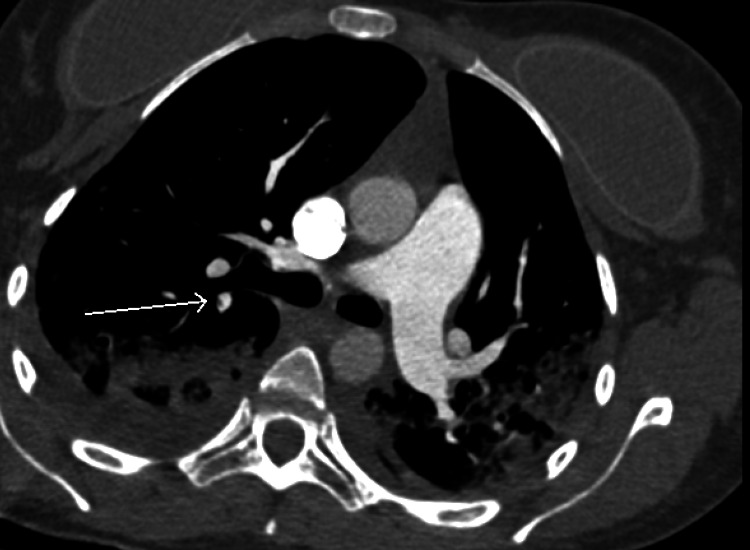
CT chest pulmonary embolism protocol demonstrating fat density branching filling defect within right upper lobe segmental pulmonary artery with new diffuse airspace disease including ground-glass opacities and interlobular septal thickening
